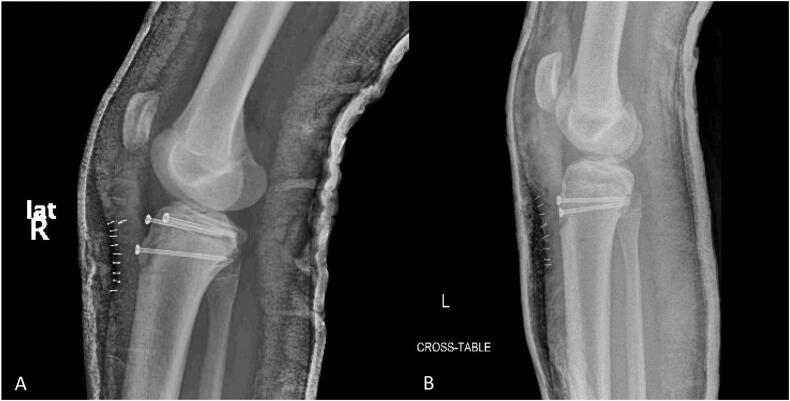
Immediate postoperative plain radiographs of both knees. (A) Right knee showing three 4.0 mm partially threaded cannulated screws. (B) Left knee showing two 4.0 mm partially threaded cannulated screws.
